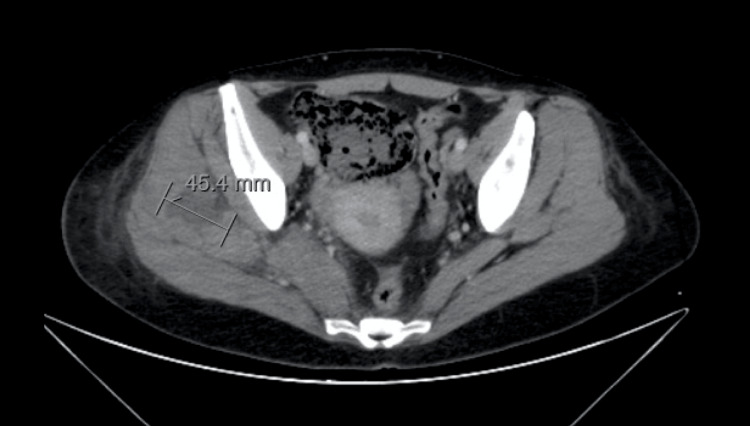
Pelvic CT scan performed on the third day. The contrasted CT scan revealed a multiloculated intramuscular collection in the right gluteal region, approximately 45 x 70 mm in size.
